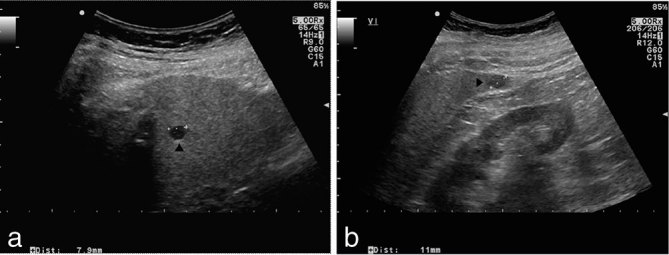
Ultrasound imaging of the liver showing a hypoechoic, well-delimited lesion in segment 8 (a, black arrowhead) and 6 (b, black arrowhead) measuring 8 and 11 mm in maximum diameter, respectively. Mild diffuse increased echogenicity of the liver parenchyma is also present, consistent with fatty infiltration.
